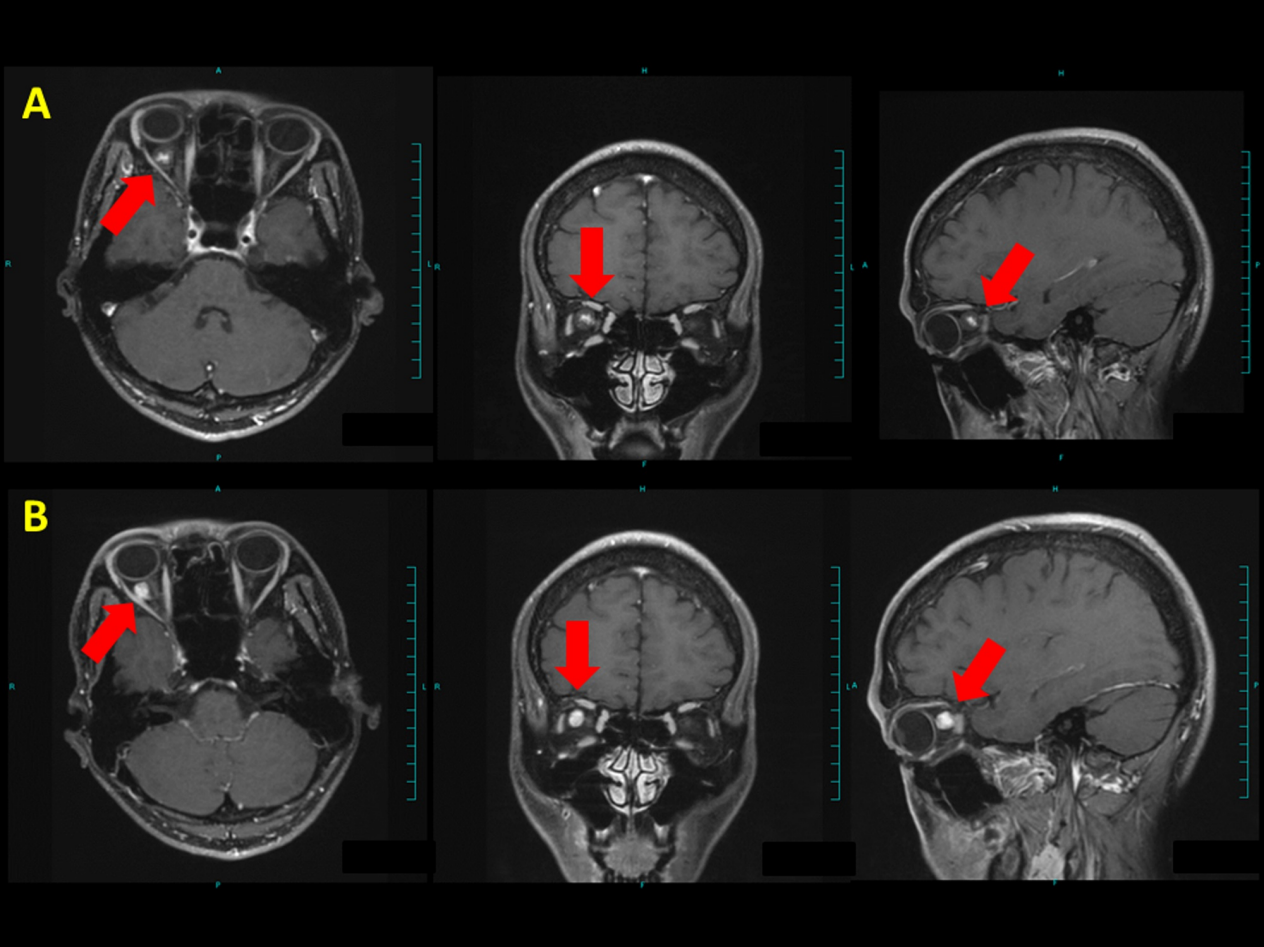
Brain MRI of case 5 (A) Before treatment and (B) 8 months after treatment. Red arrows denote tumor. The tumor decreased in size.
